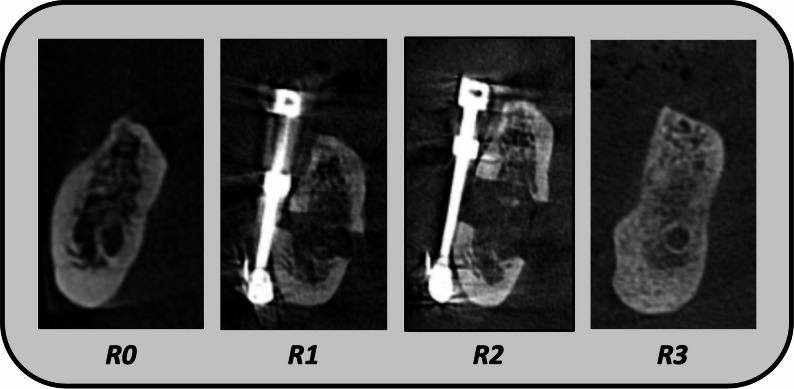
A complete description of the radiographic timeline of the study. R0, Preoperative CBCT. R1, pre-activation CBCT. R2, Post-Activation CBCT. R3, Post-Consolidation CBCT
